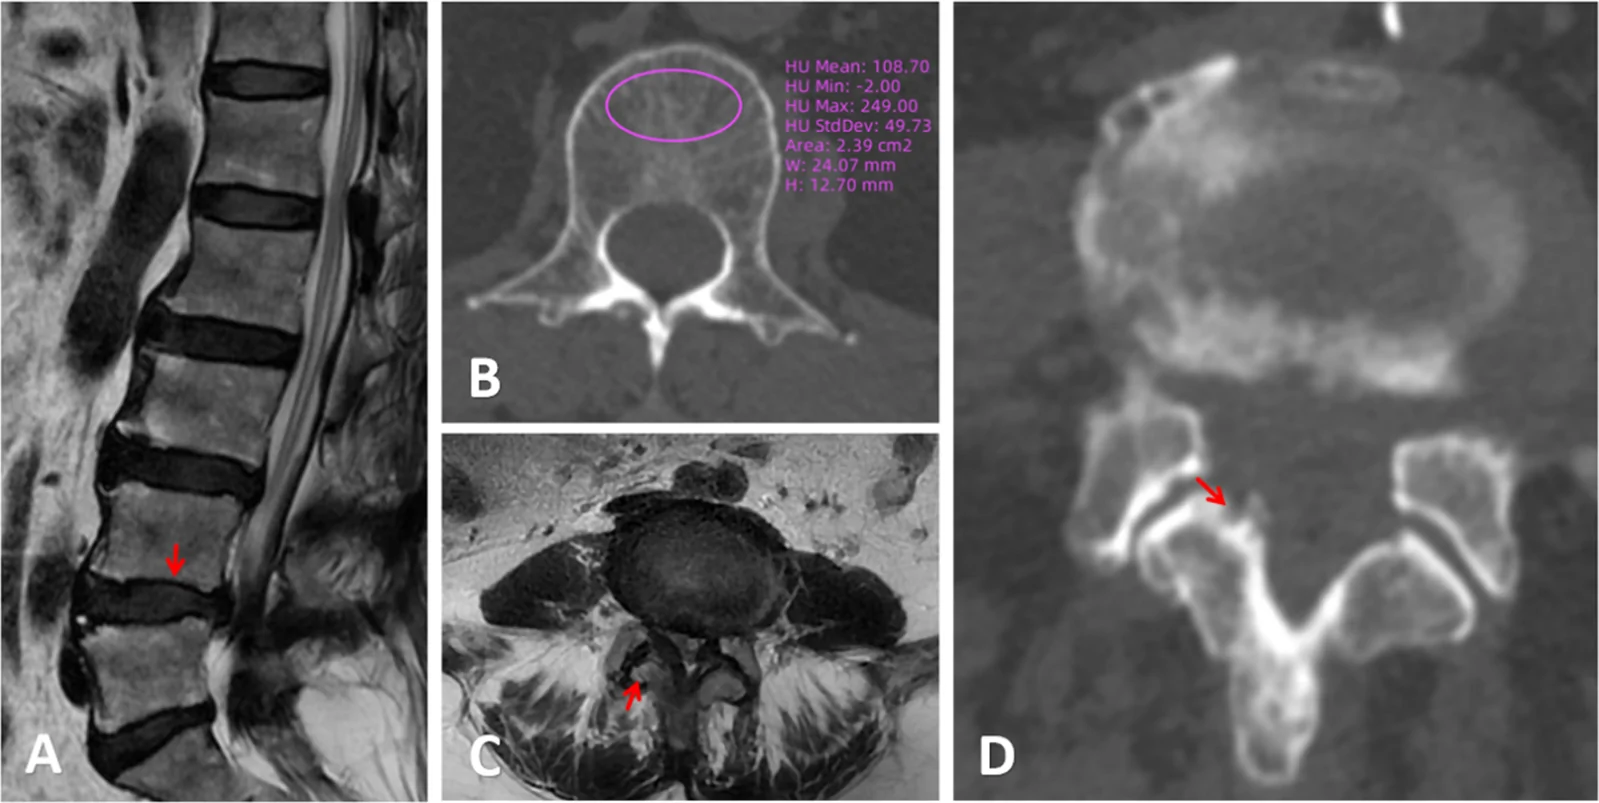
(A) T2-weighted MRI shows grade IV degeneration of the L4-5 intervertebral disc. (B) Axial CT image at the mid-portion of the L1 vertebral body shows an anterior column CT-HU value of 108.70. (C) Axial MRI reveals severe stenosis at the affected level, with grade III facet joint degeneration. (D) The facet joints at the affected level are classified as grade III.
